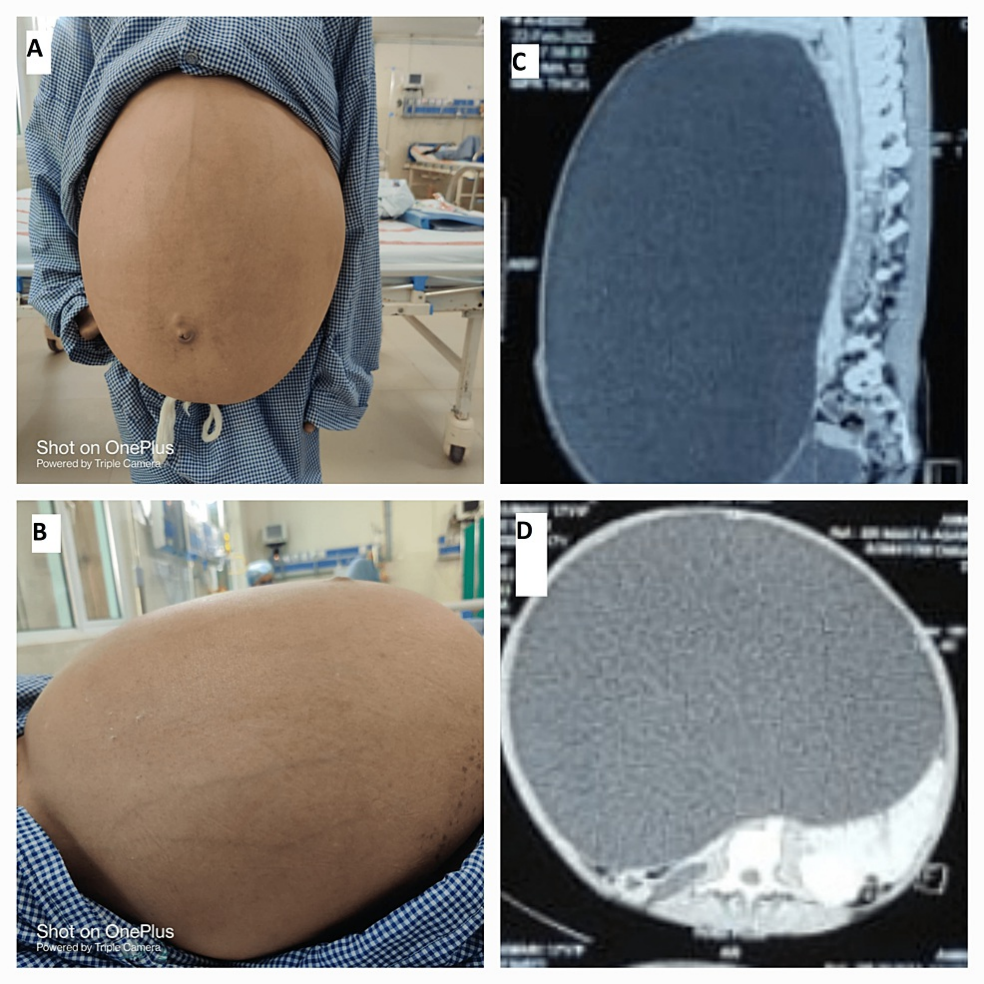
(A) Front view of patient’s abdomen before surgery. (B) Lateral view of patient’s abdomen before surgery. (C) CT imaging showing sagittal sections of giant ovarian cyst. (D) CT imaging showing axial sections of giant ovarian cyst.
